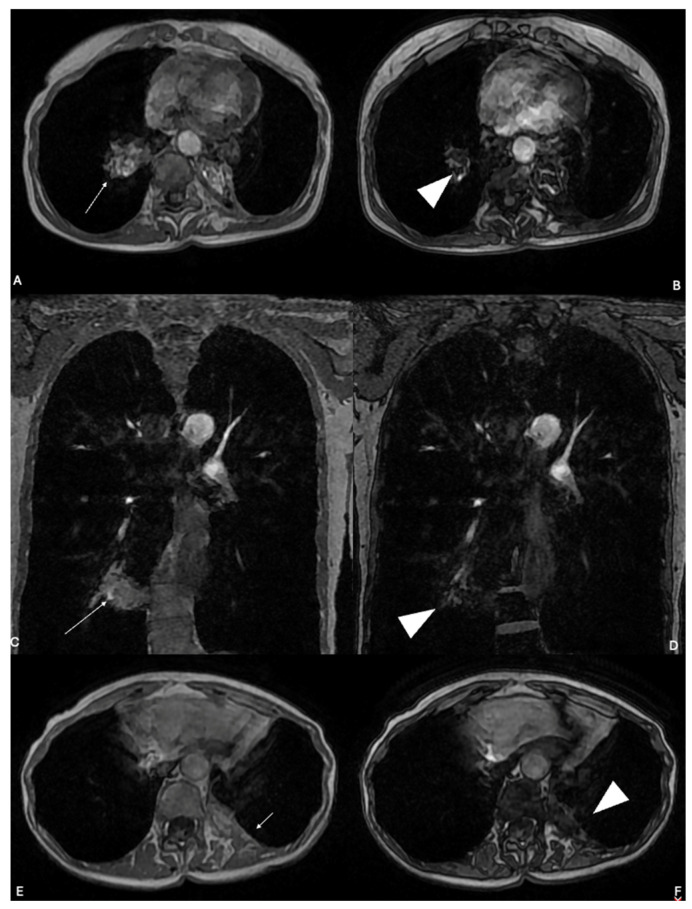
Chemical-shift chest MRI with paired in-phase and opposed-phase (out-of-phase) gradient-echo images: axial in-phase (A) and corresponding axial opposed-phase (B); coronal in-phase (C) and corresponding coronal opposed-phase (D); additional axial in-phase (E) and corresponding axial opposed-phase (F). Focal pulmonary lesions (arrows) show a conspicuous signal drop on opposed-phase images compared with in-phase images, consistent with intracellular lipid. Arrowheads indicate the corresponding opposed-phase signal loss/chemical-shift (“India ink”) effect at lesion margins, supporting fat-containing inflammatory deposits in the appropriate clinical context of lipoid pneumonia.
